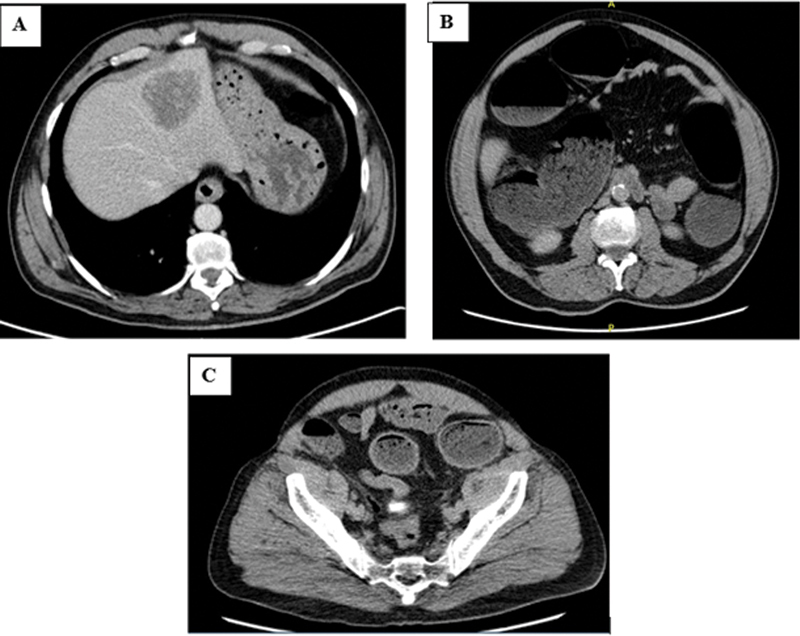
Abdominal enhanced computed tomography scan showing: (
